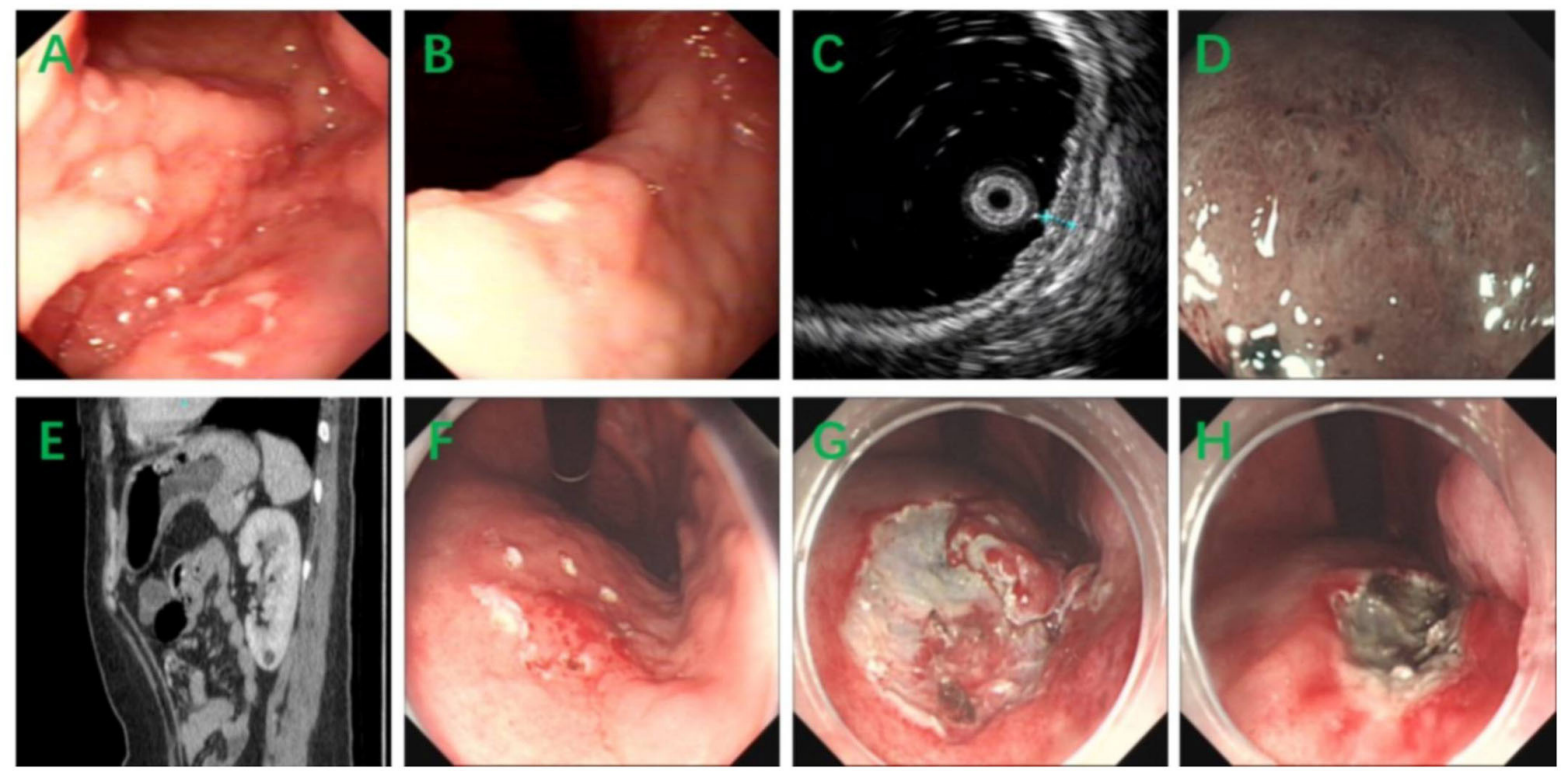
(A,B) Gastroscopy revealed multiple erosion and ulcer in gastric body and gastric angle. (C) EUS revealed hypoechoic thickening of the mucosa layer. (D) ME-NBI showed irregular marginal crypt epithelium and subepithelial capillary network. (E) Abdominal computed tomography showed thickening of the wall of gastric body and gastric fundus and mild enhancement, and the surrounding lymph nodes were enlarged. (F–H) The procedure of ESD.
